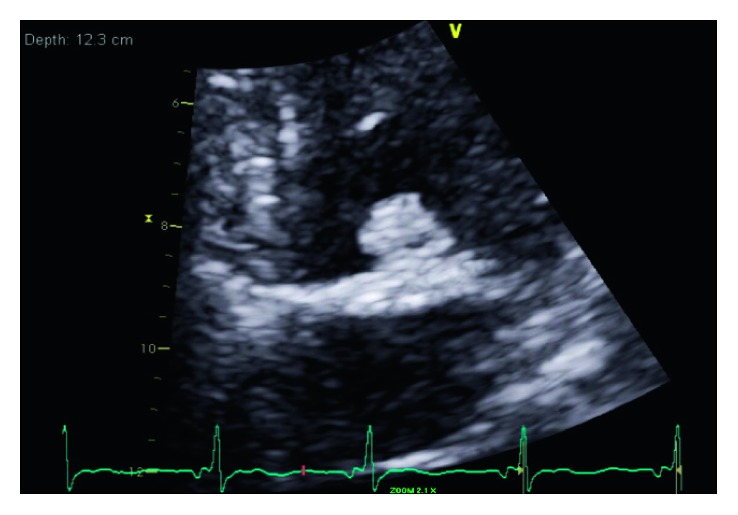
Transthoracic echocardiogram (TTE) showing a 1.2 × 1.2 cm mobile mass attached to the ventricular aspect of mitral valve and hyperechogenic mitral annulus.
